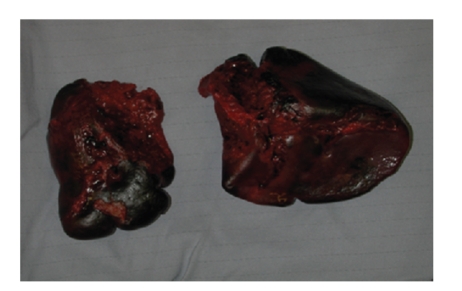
Case I: Appeared intact on CT but avulsed and in 2 pieces at laparotomy.
